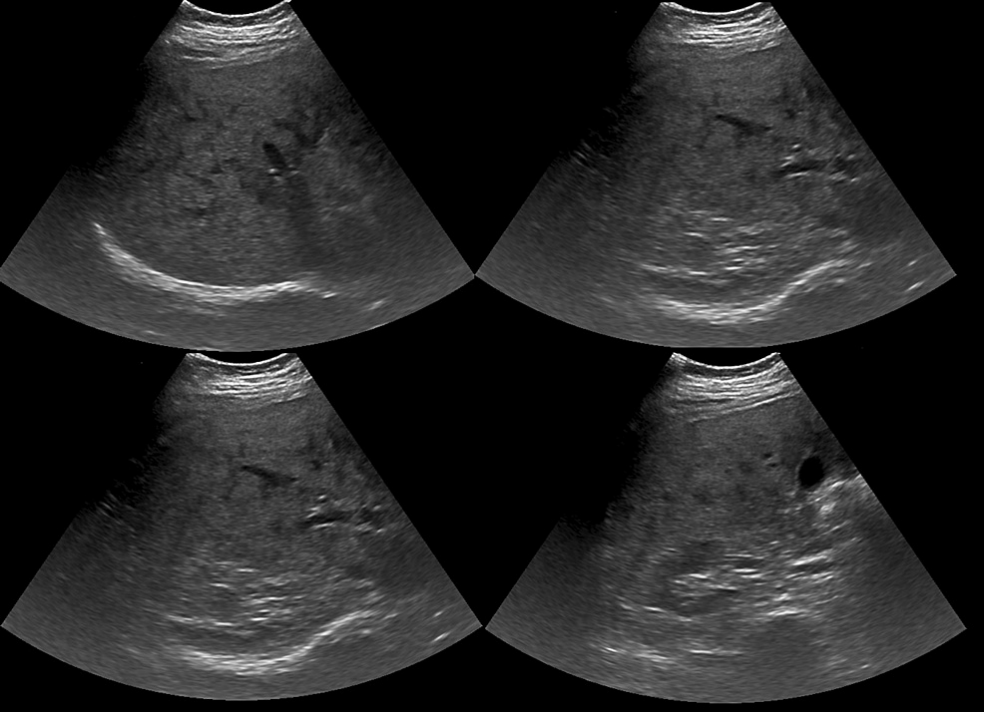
Ultrasound of the liver showing an enlarged liver measuring 17.0 cm with nodular and irregular contour. The parenchyma appears heterogeneous with diffusely increased echogenicity.
